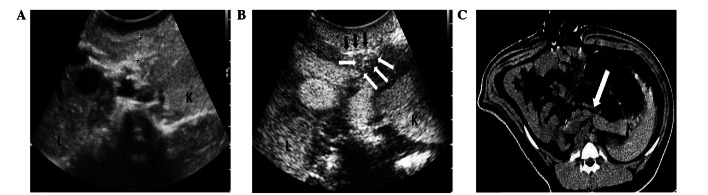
Images of a deep laceration. (A) A deep laceration of the pancreas was not clearly shown in the US image. The parenchyma shown between the plus signs was inhomogeneous. (B) The laceration (white arrow) was identified as inhomogeneous with high enhancement by CEUS. The accumulation of the contrast agent was shown as hyperechoic in the interstice between the pancreas and bursa omentalis (black arrows). (C) The laceration was hypointensive in the CECT image. K, kidney; L, liver; US, ultrasound; CEUS, contrast-enhanced ultrasonography; CECT, contrast-enhanced computed tomography.
